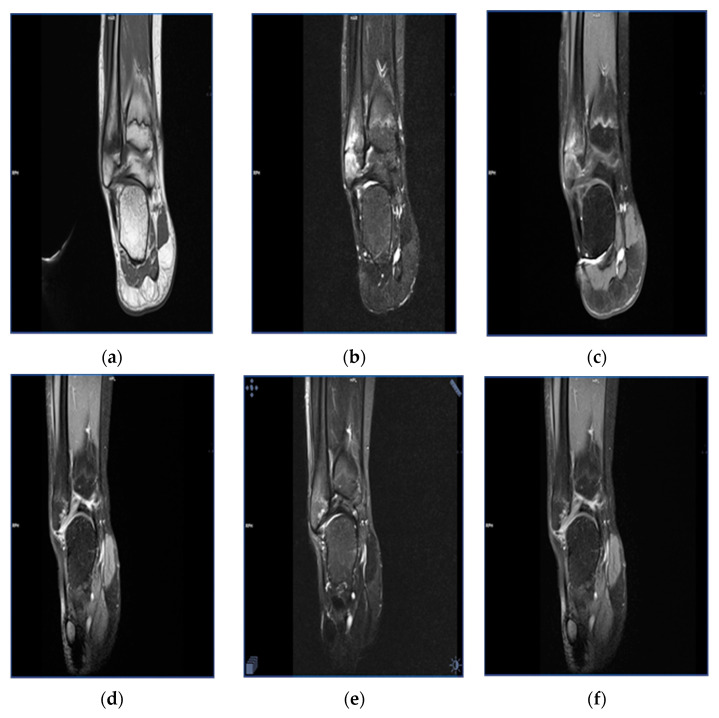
Magnetic resonance images in a 13-year-old female patient with bone marrow edema within the head of the fibula. Status before treatment: T1-weighted sequence (a), T2-weighted sequence (b), T1-weighted sequence with contrast enhancement (c). Status after a 15-month follow-up, with regression of the bone marrow edema: T1-weighted sequence (d), T2-weighted sequence (e), T1-weighted sequence with contrast enhancement (f).
